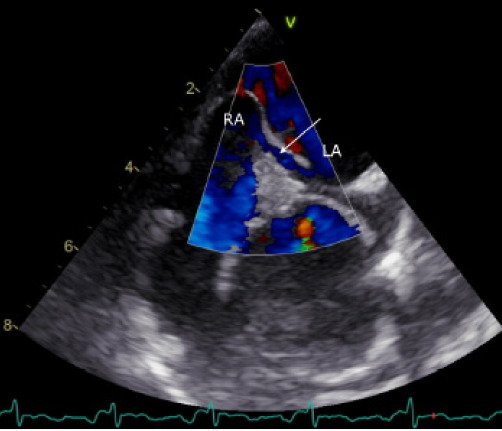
Transesophageal echocardiography, color Doppler mid-esophageal bicaval view. A long-tunnel patent foramen ovale (PFO) is seen with flow directed from the right atrium (RA) to the left atrium (LA). A white arrow marks the color Doppler jet across the PFO tunnel.
